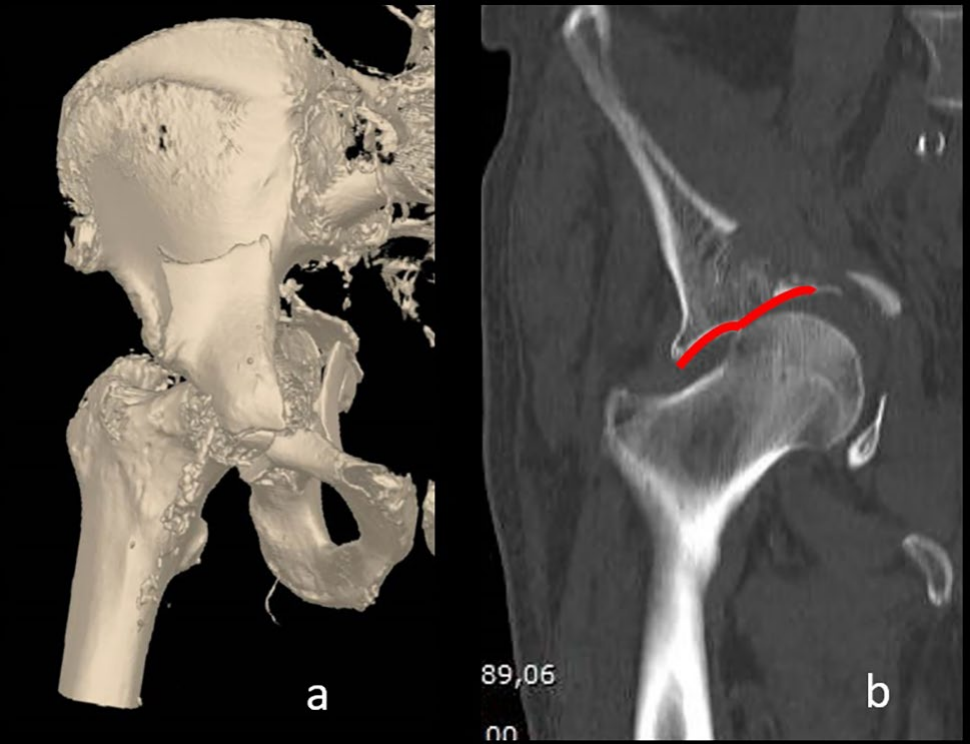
a A 3D reconstruction of a two columns fracture with the involvement of the quadrilateral plate occurred in a 75-year-old male. b Coronal reconstruction of a CT scan. Please note in red the “gull sing” that represent the result of the supero-medial impaction of the femoral head
